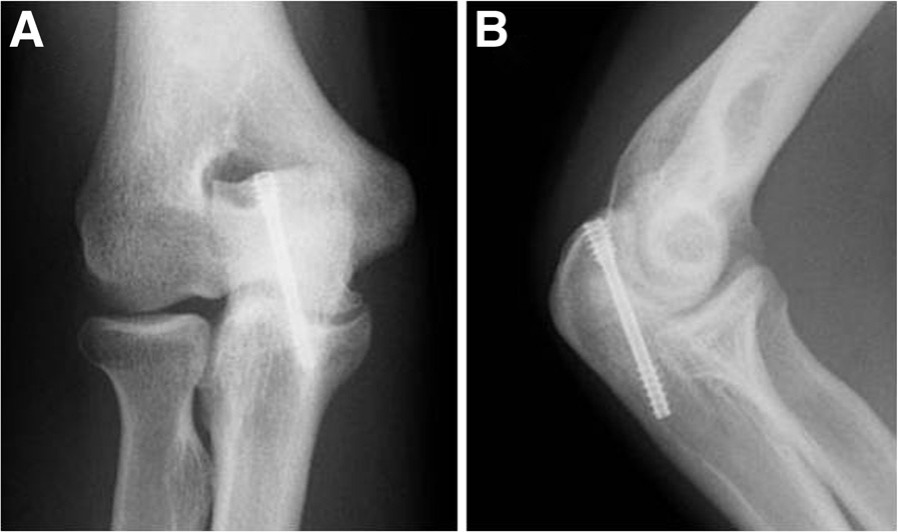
Case 2. Radiographs after surgery. The screw was inserted through a small incision with neither fracture site open nor bone graft and the fracture site was fixed with the screw on anteroposterior (A) and lateral (B) views of the radiographs. The distal thread of the screw reached the medial cortex of the ulna and the proximal thread was located in the cancellous bone of the olecranon.
